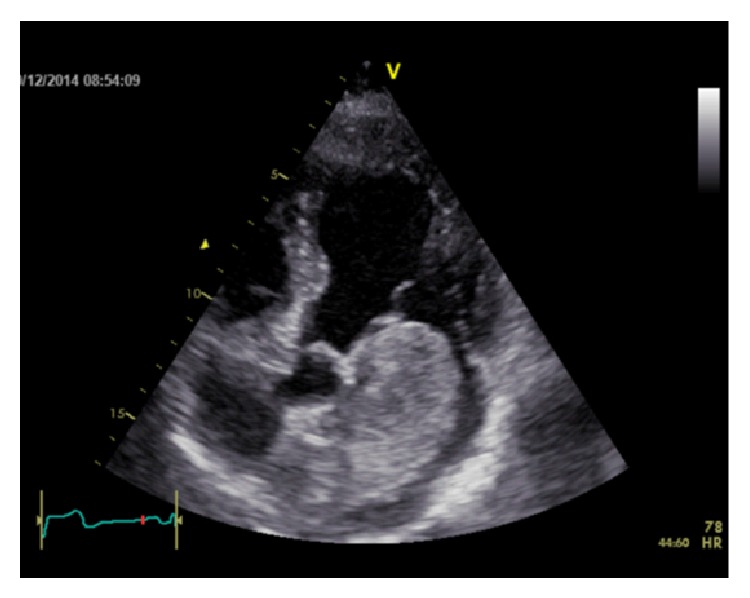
Transthoracic echocardiography shows the giant left atrial myxoma protruding through the mitral valve into the left ventricle during diastole.
